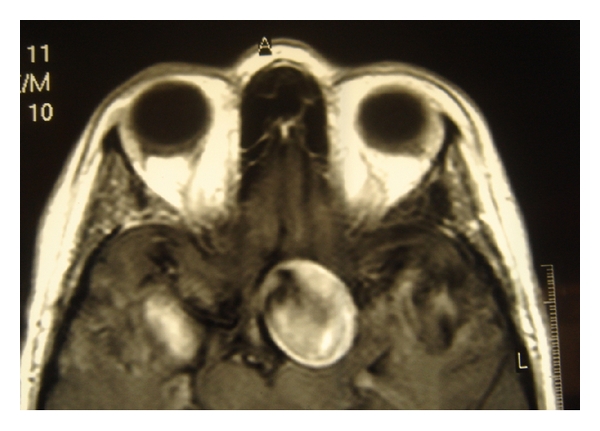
Axial T1-weighted MRI revealing the giant internal carotid aneurysm.
